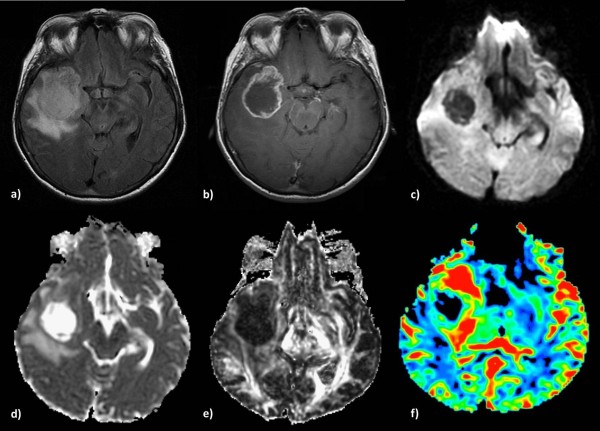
Glioblastoma multiforme in a 65-year-old woman. Axial T2-weighted (a) and T1-weighted post contrast (b) images demonstrate a right temporal lesion with surrounding edema and ring-shaped enhancement. On the DW-image the lesion presents low signal intensity (c) resulting in higher intratumoral ADC (d), lower intratumoral FA (e), and high peritumoral rCBV (f), reflecting tumor infiltration in the surrounding parenchyma.
